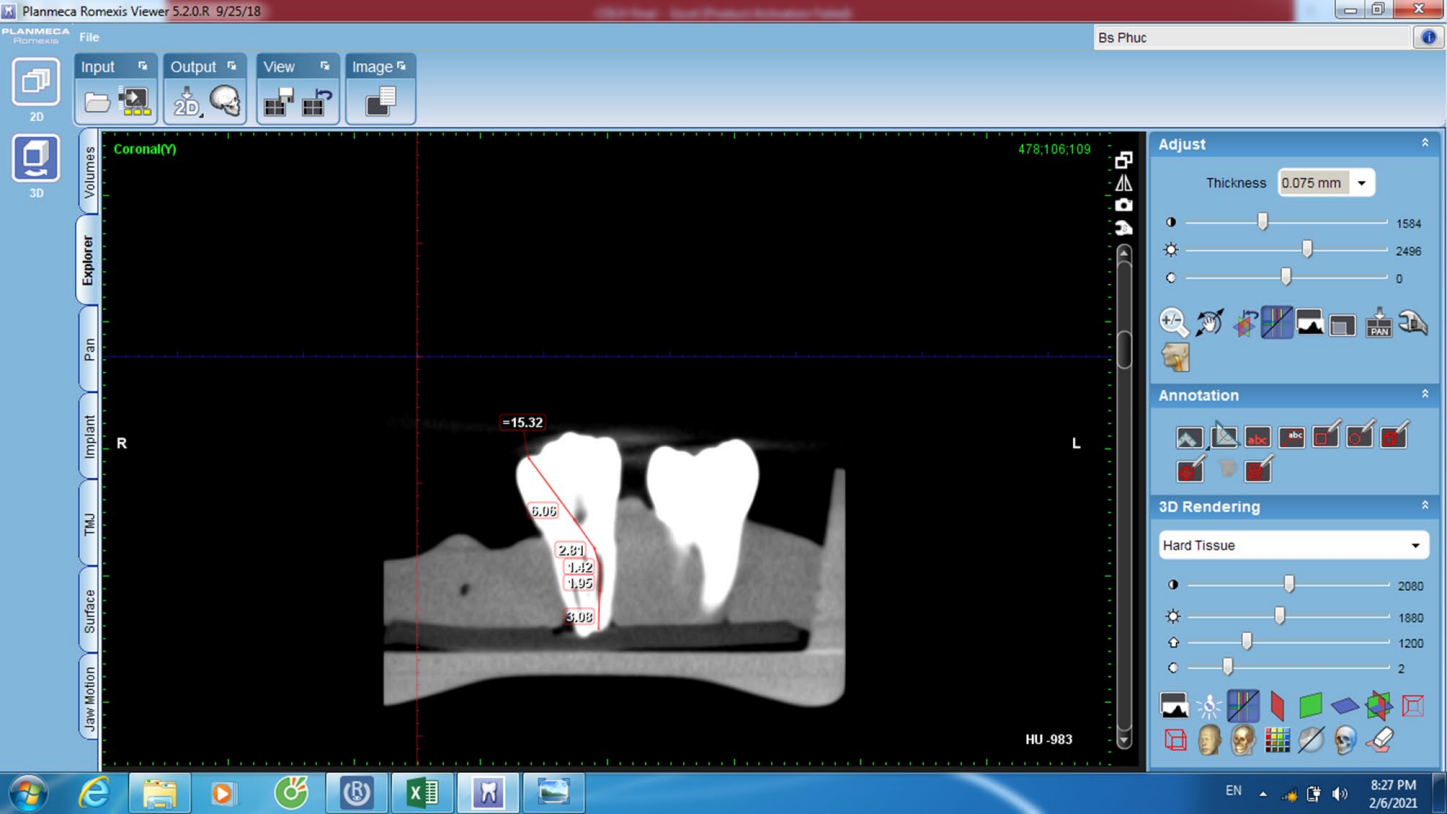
Romexis Viewer measurement on the conventional CBCT software.
